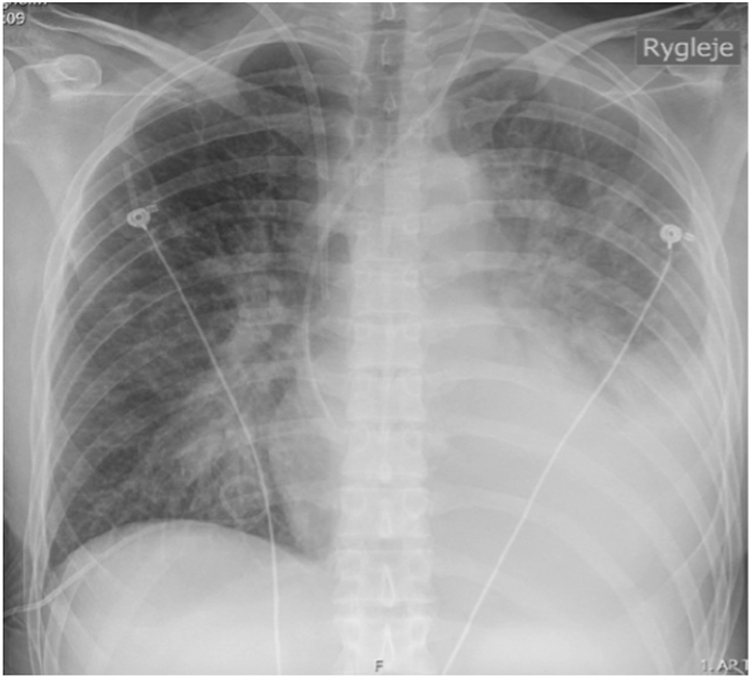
Chest radiograph with Kerley B lines and increased cardiothoracic ratio.
